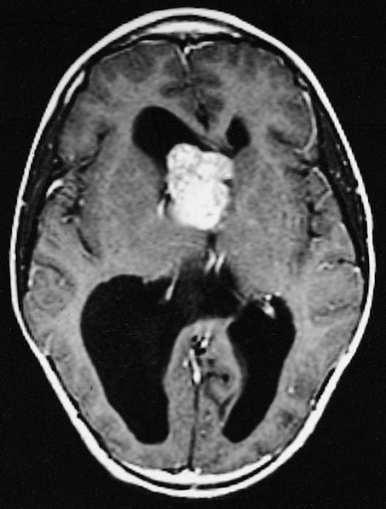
MRI of a SEGA (Image courtesy of Wikimedia Commons [online]. Website https://commons.wikimedia.org/wiki/File:MRI_of_brain_with_subependymal_giant_cell_astrocytoma.jpg [accessed 05 January 2020].).
